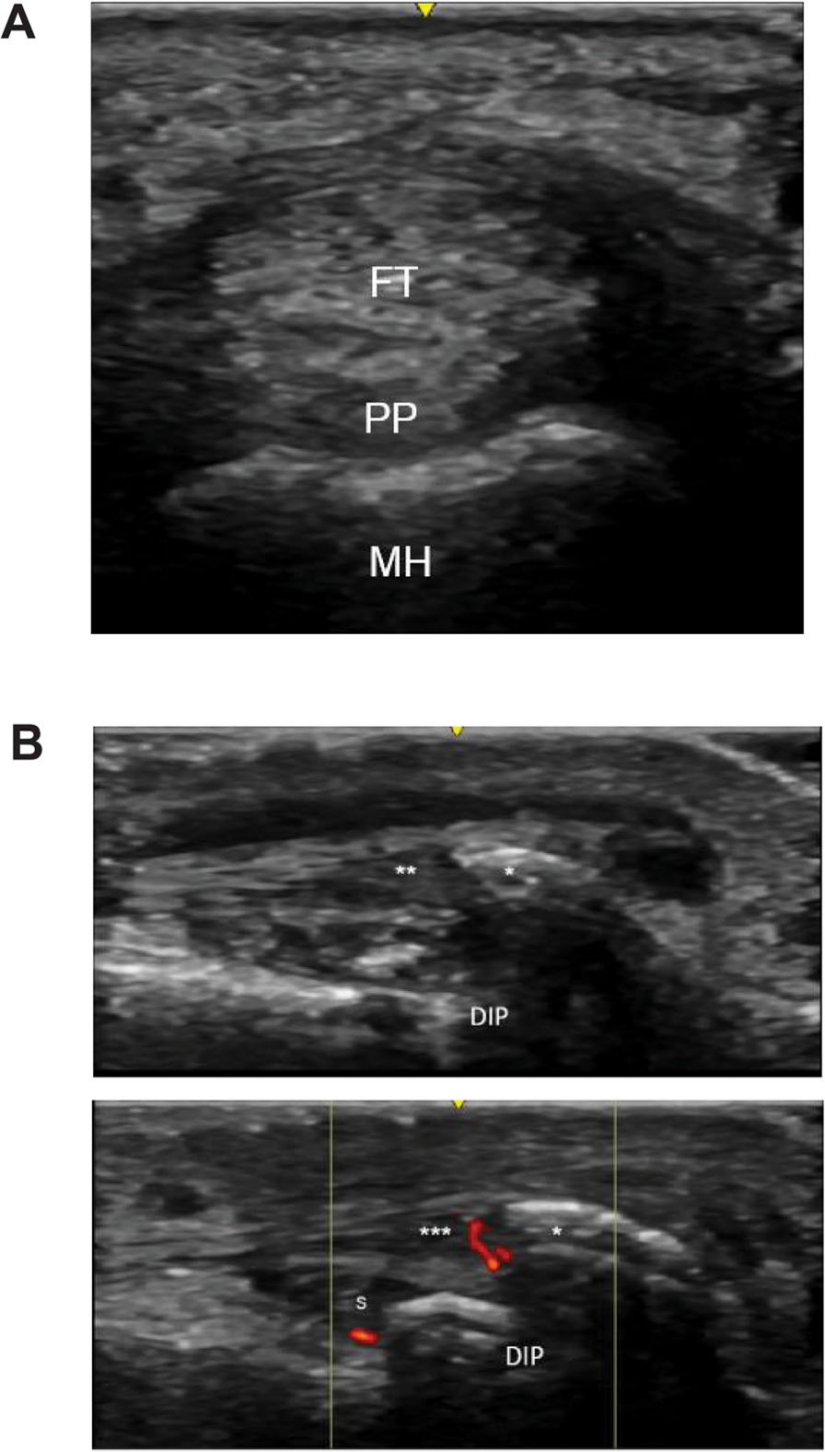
Ultrasound findings for differentiation of psoriatic arthritis from rheumatoid arthritis. a Short-axis view of palmar plate inflammation. FT, flexor tendon; MH, metacarpal head; PP, palmar plate. b Dorsal long view of enthesitis of the extensor tendon from a distal interphalangeal joint in a patient with psoriatic arthritis. DIP, distal interphalangeal; S, DIP synovitis; asterisk (*), enthesophyte; double asterisks (), extensor tendon demonstrating thickening, hypoechogenicity, and loss of fibrillar architecture; triple asterisks (*), extensor tendon with insertional Doppler
